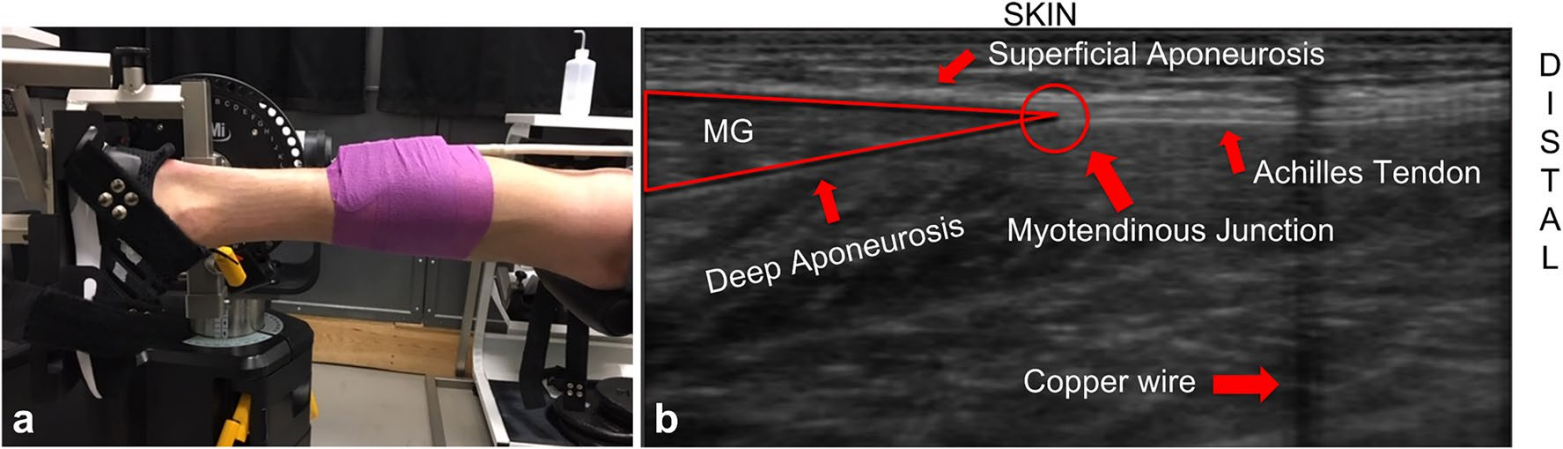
(a) Positioning of the participant in the Cybex with the ultrasound probe attached. (b) Labelled ultrasound image when probe was secured to the participant.
